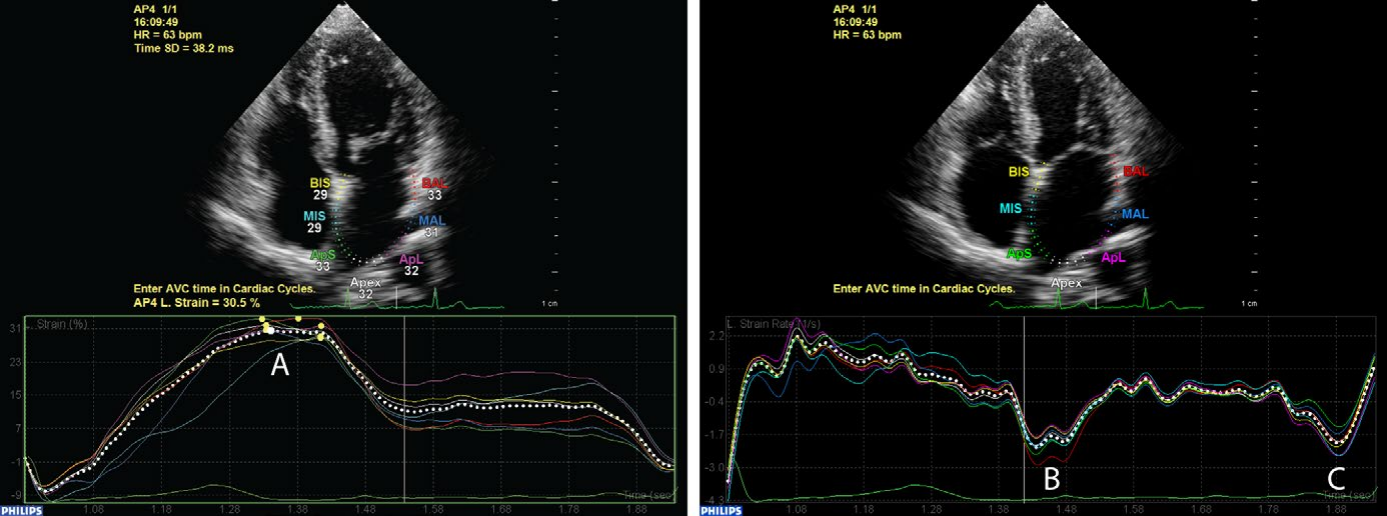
Example of left atrial (LA)‐strain measurement in a apical four‐chamber view. LA‐strain(A) is measured as the maximum strain value during atrial diastole. Conduit (B) and pump (C) function are measured using strain rate
